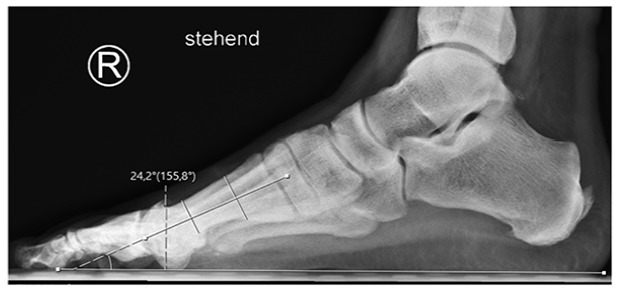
Elevation angle (ELEA) of the anatomical axis of the first metatarsal bone in relation to a line parallel to the ground assessed on a mediolateral radiograph in a standing position with full weight-bearing.
